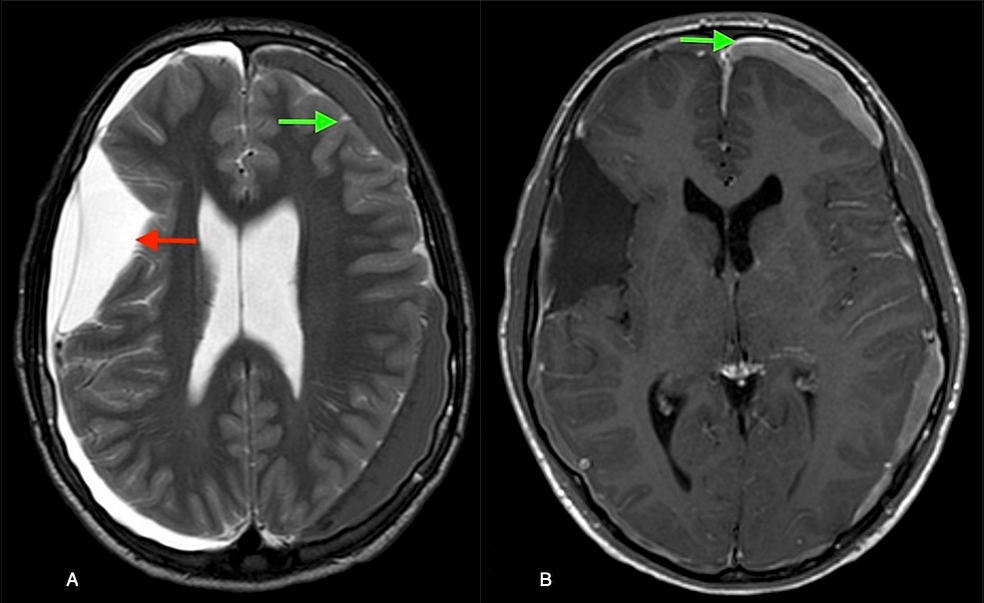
Axial T2 MRI brain (3A) showing decreased arachnoid cyst (red arrow) and subdural hematoma (green arrow). Contrast-enhanced axial sequence (3B) showing pachymeningeal enhancement (green arrow).
